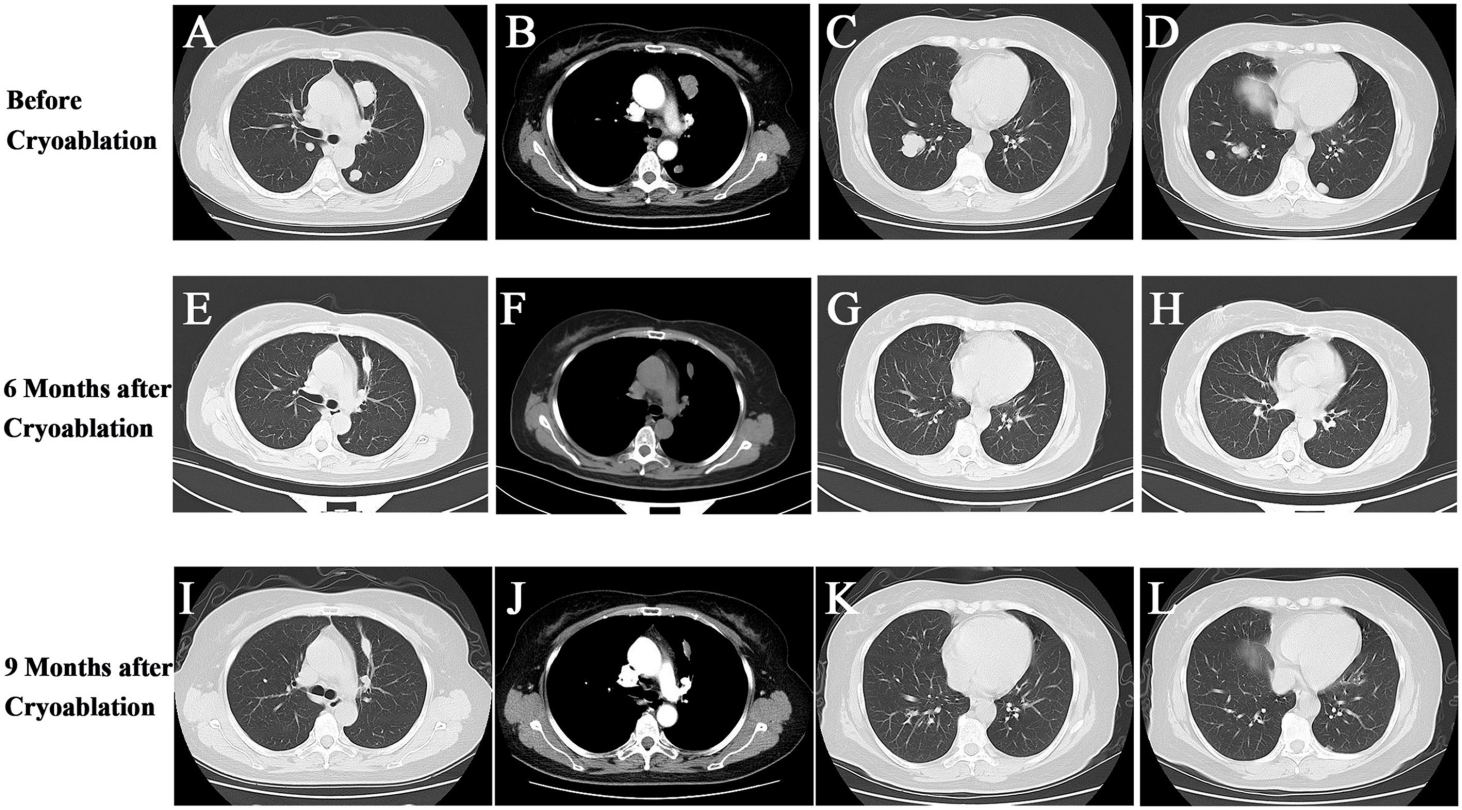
1 CT follow-up images of the patient before and after cryoablation. (A–D) Before cryoablation of the patient, multiple masses and nodules of variable size were seen in the left upper lobe and lower lobe of both lungs. (E–H) Six months after cryoablation of the more significant lesion in the left upper lobe, the frozen area was seen to be smaller and firmer; the remaining multiple lung lesions had largely disappeared. (I–L) Nine months after cryoablation, only scarring was seen in the cryoablation area, and no lesions were seen in both lungs.
